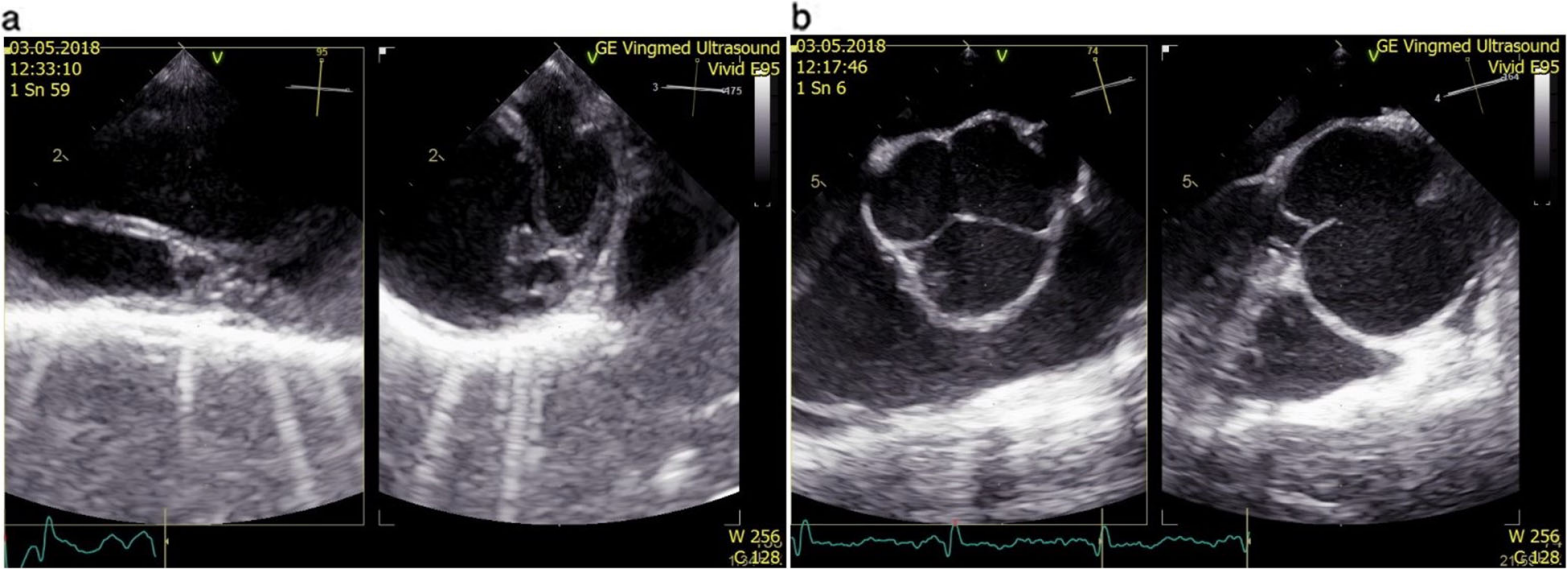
a Transesophageal echocardiography showing an intimal flap through the whole length of the descending aorta with a small mobile echogenic structure in the proximal part of the false lumen b Dilated aortic root and tricuspid aortic valve
